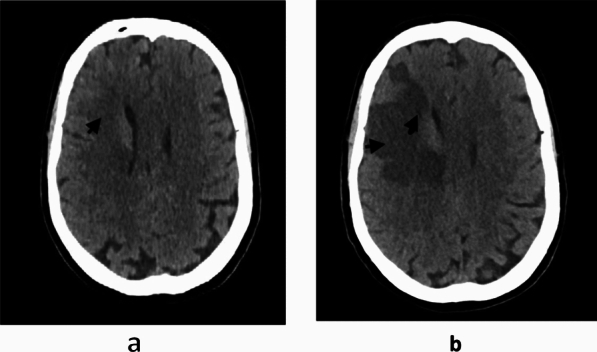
a Brain CT scan taken 8 h after left-sided hemiplegia; the arrow indicates early ischemic changes. b Control brain CT scan taken 72 h after left-sided hemiplegia; arrows indicate extensive cortical infarct
